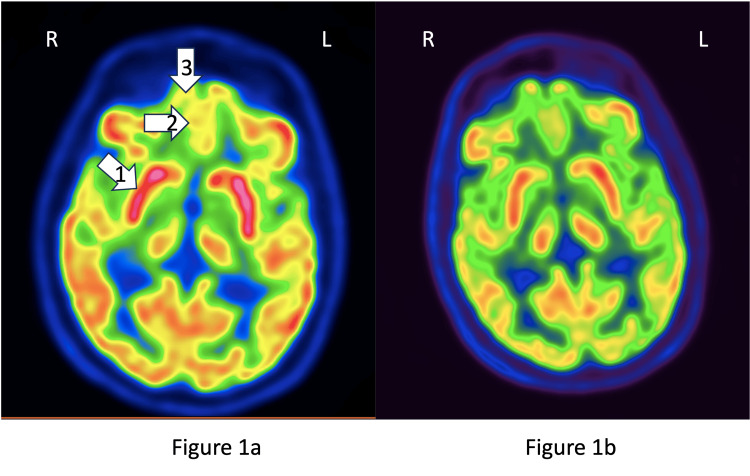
PET FDG Scans In a PET scan, colors showing decreasing order of metabolic activity are red, yellow, green, and blue. 1a shows areas of hypermetabolism, indicated by red/red-white shade for the basal ganglia (1), yellow shade for the olfactory cortex (2), and superior/middle frontal gyri (3) leading to the diagnosis of autoimmune encephalitis. Following the five-day course of IVIG, these areas (1b) showed hypometabolism (trending towards normal) as indicated by a decrease in the intensity of red shade for basal ganglia (1), green shade for olfactory cortex (2), and green-yellow shade for superior and middle frontal gyri (3). PET FDG: Positron emission tomography fluorodeoxyglucose
